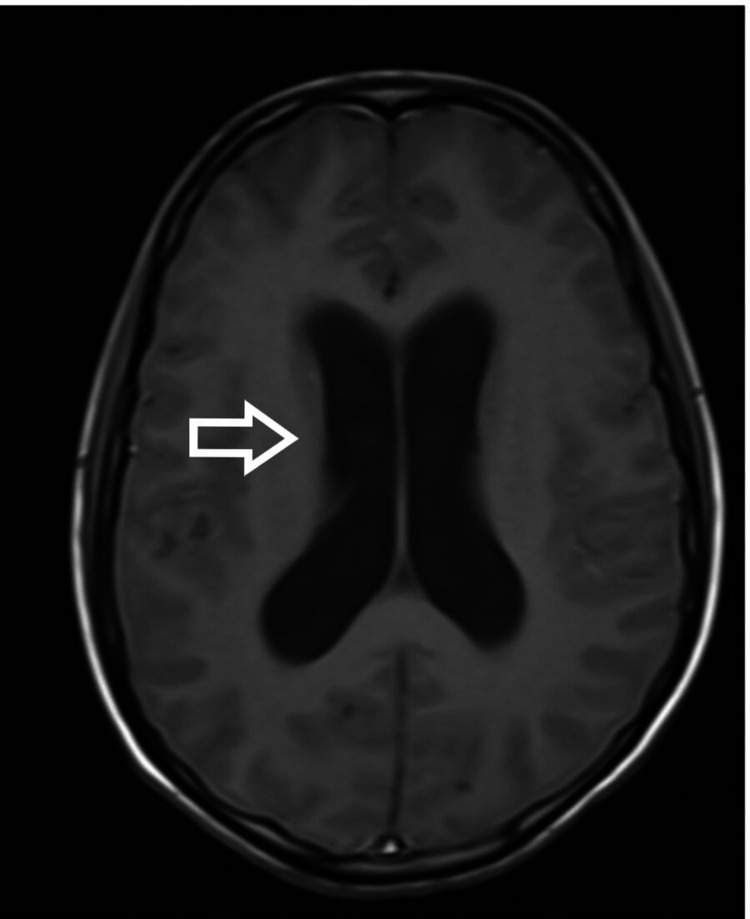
MRI brain showing evidence of hydrocephalus with leptomeningeal enhancement on the post-contrast image - view 1 MRI: magnetic resonance imaging
